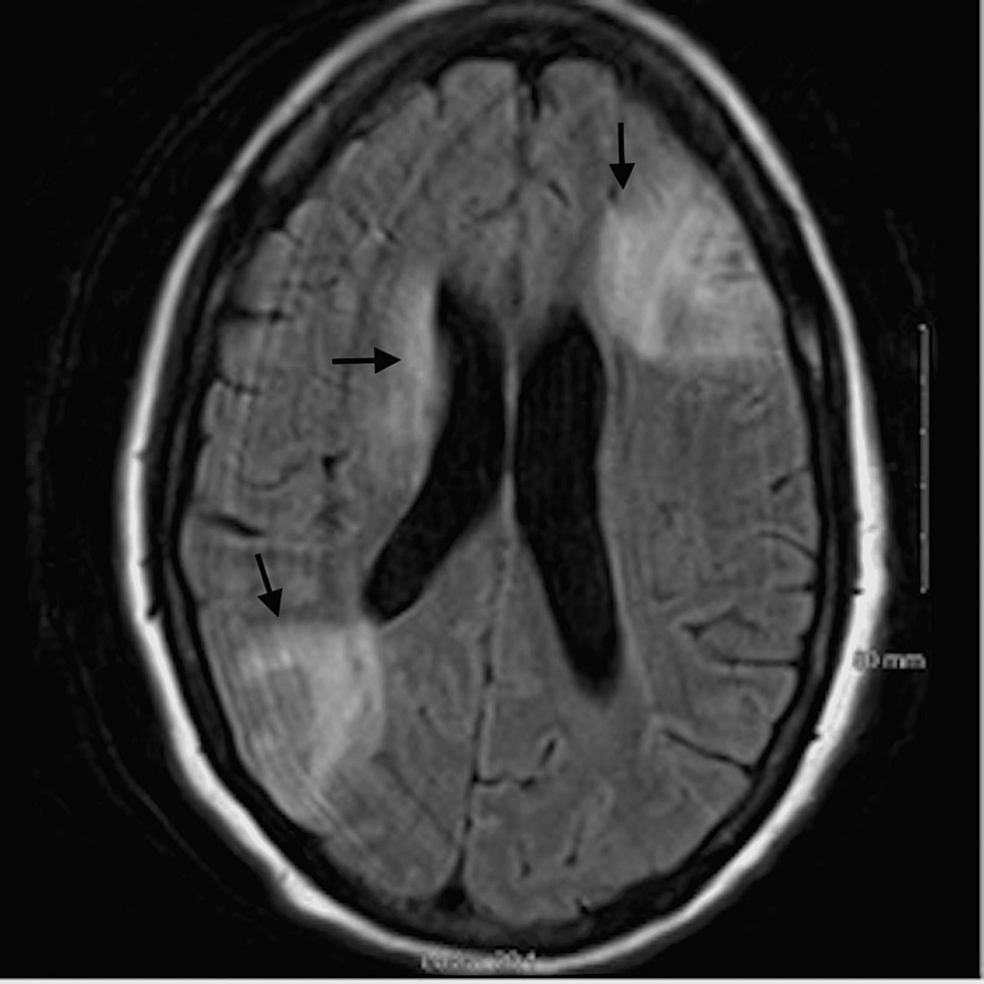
MRI Brain MRI image showing patchy acute infarctions with associated petechial hemorrhage and areas of leptomeningeal enhancement within the left frontal lobe and parietal lobe marked with arrows.
